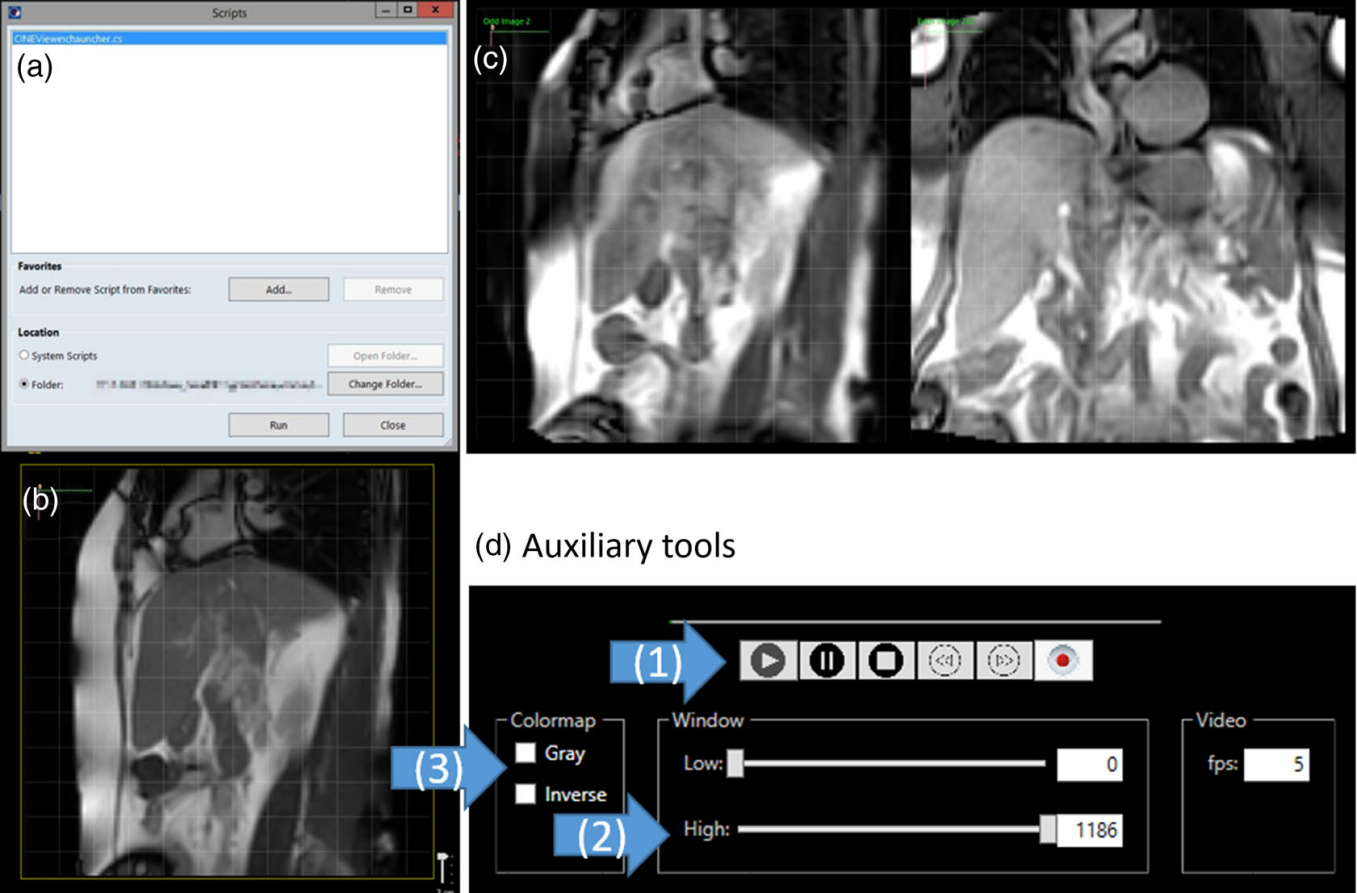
User interface of Cine Viewer in Eclipse. (a) Eclipse script window, (b) single display panel for sagittal cine MRI, and (c) dual display panel for alternating sagittal and coronal cine MRIs. (d) Auxiliary tools include (1) the image playback mode, (2) the image contrast adjustment, and (3) the color map selection
